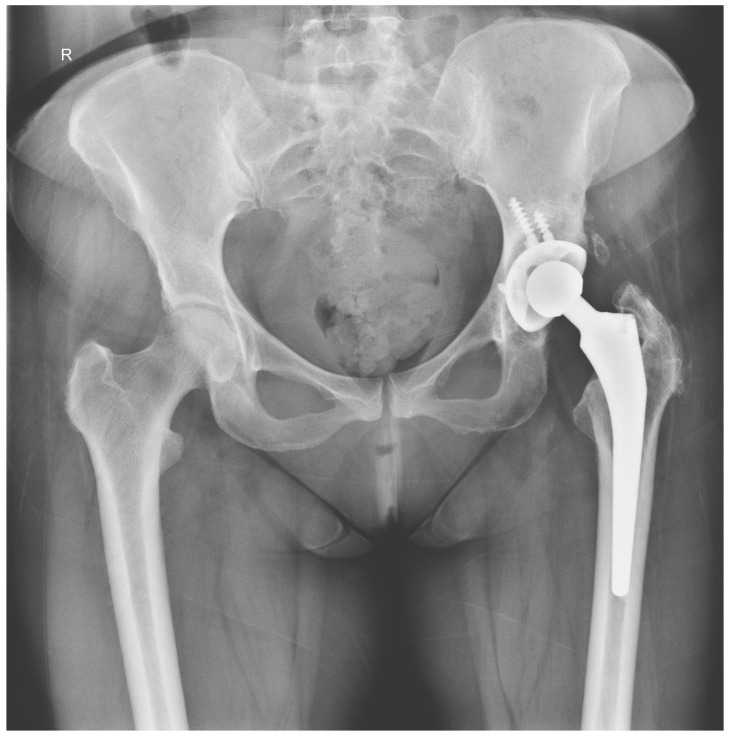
X-ray of pelvis, total left hip Arthroplasty.
